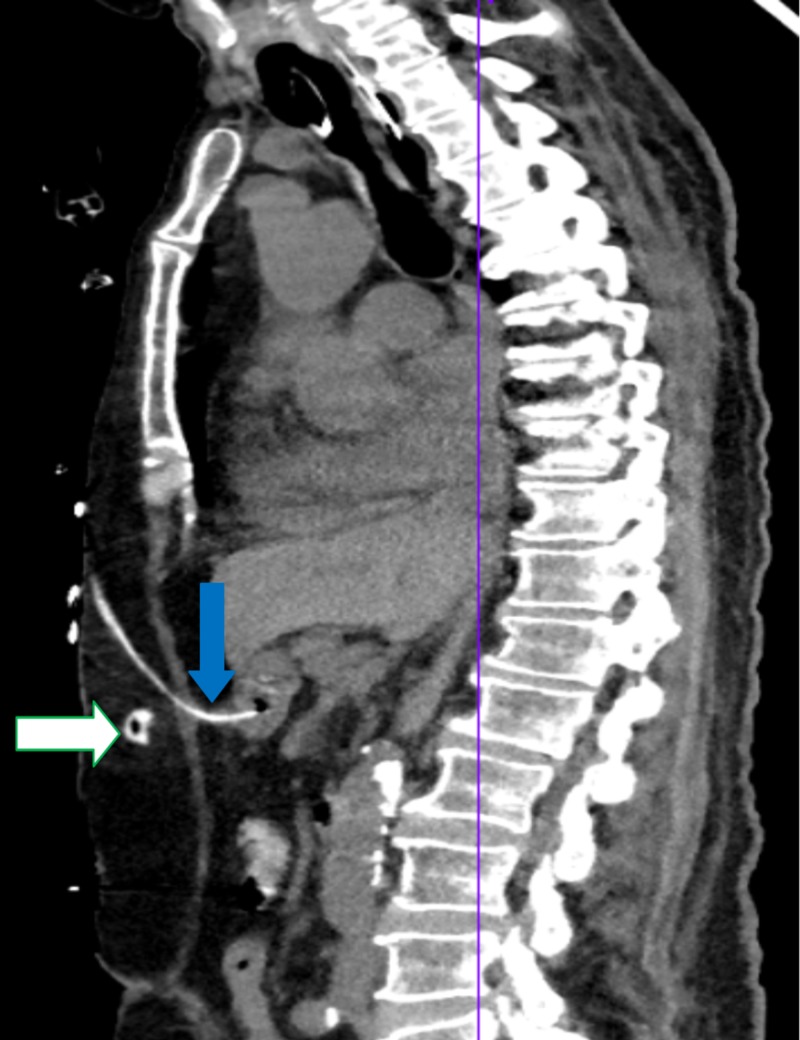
CT of the abdomen/pelvis: VP shunt terminating inside gastric lumen (blue arrow) alongside displaced PEG tube (white arrow) CT, computed tomography; VP, ventriculoperitoneal; PEG, percutaneous endoscopic gastrostomy
